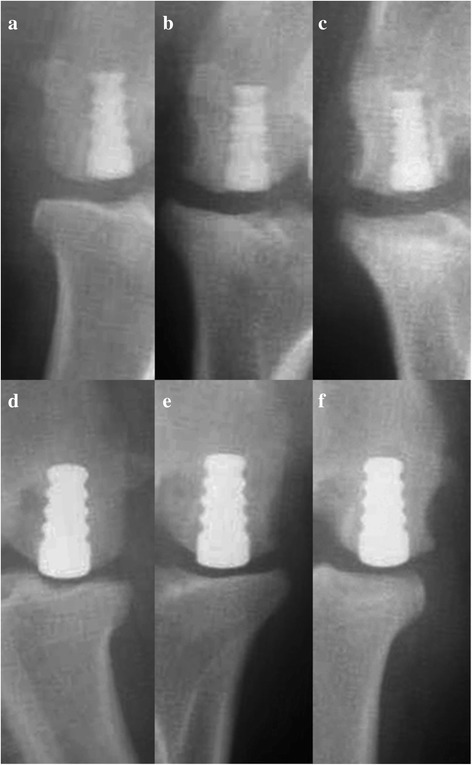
Postoperative radiographs: pyrolytic carbon (*top*) and Co-Cr (*bottom*) implants at 12 weeks (a, d), 24 weeks (b, e), and 52 weeks (c, f)
